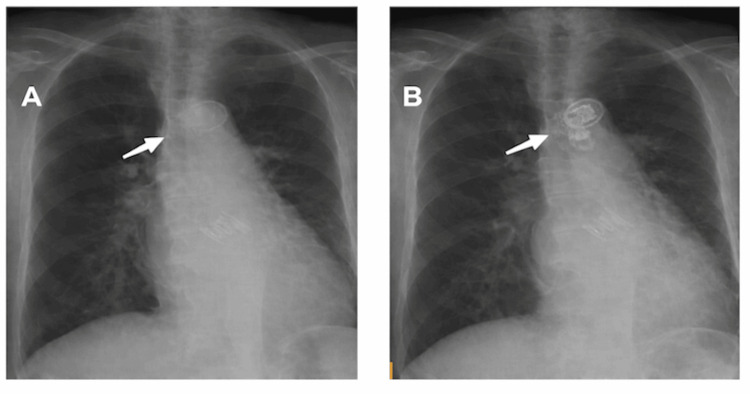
Chest radiographs demonstrating valve-in-valve-in-valve configuration. (A) Pre-procedural chest radiograph demonstrating the previously implanted balloon-expandable transcatheter aortic valve in the aortic position. (B) Post-procedural chest radiograph obtained after implantation of the third valve, demonstrating the valve-in-valve-in-valve (ViViV) configuration. Arrows indicate the location of the prosthetic valve complex within the aortic root.
